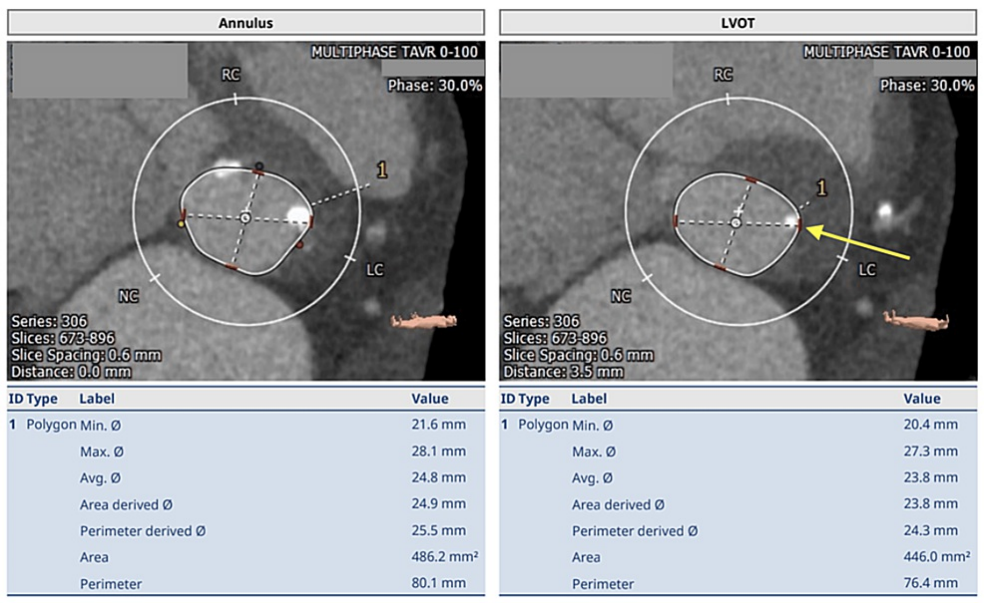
TAVR protocol computed tomography (CT) scans demonstrated no significant aortic annular calcification; however, the presence of a calcified nodule can be seen in the annulus shown in the left panel, which is extending into the LVOT shown in long axis view in the right panel indicated by the yellow arrow. LVOT: left ventricular outflow tract; TAVR: transcatheter aortic valve replacement
